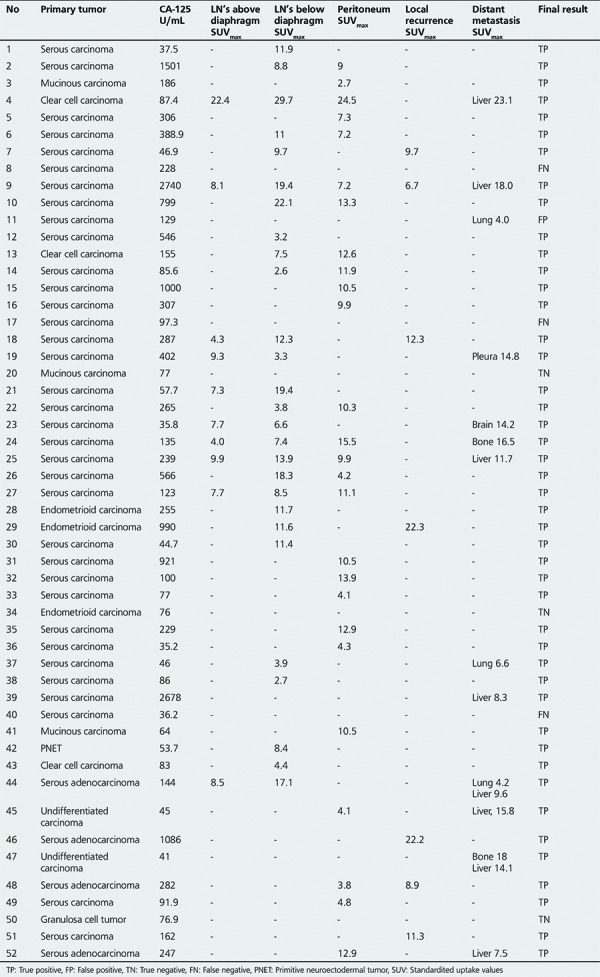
Positron emission tomography/computed tomography imaging findings and final diagnosis of all patients
